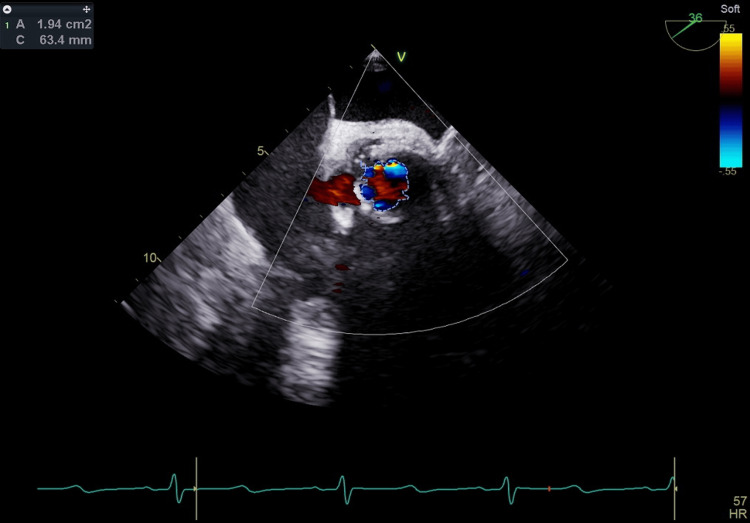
Assessment of the effective orifice area calculated by tracing the regurgitant orifice area on the aortic valve short-axis view of TEE after CPB It revealed an effective orifice area of 1.94 cm² by tracing the regurgitant orifice area on the aortic valve short-axis view of TEE. TEE: transesophageal echocardiography; CPB: cardiopulmonary bypass
